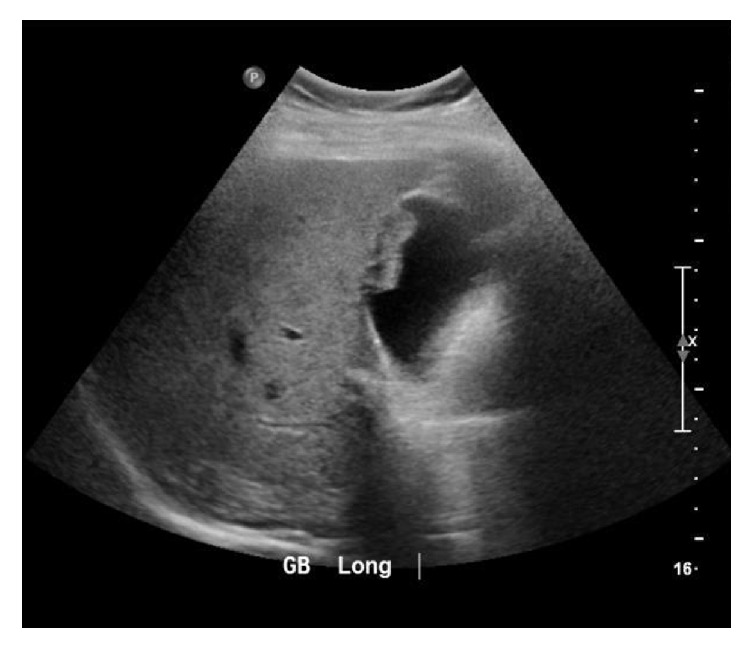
Ultrasound of the gallbladder demonstrating an irregular lesion along the gallbladder wall measuring 2.9 × 1.0 cm.
